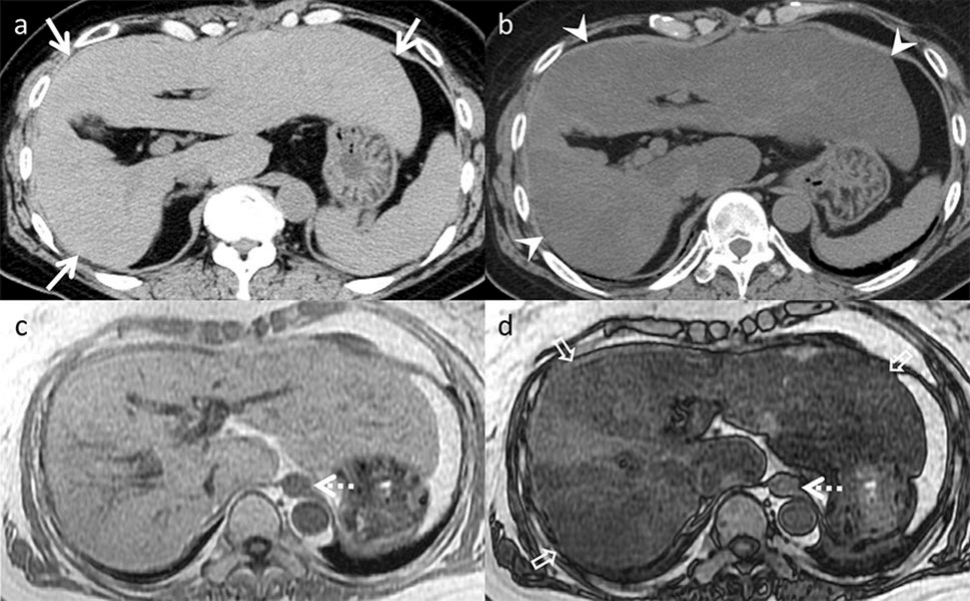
Chemotherapy-associated steatohepatitis in a 62-year-old woman with ascending colon cancer, who was undergoing chemotherapy (CTX), including irinotecan for left adrenal metastasis (dotted arrow). a Unenhanced computed tomography (CT) demonstrates normal liver attenuation (CT value, 55 HU) before CTX (arrows). b Decreased liver attenuation (21 HU) is observed on unenhanced CT performed seven months after CTX initiation (arrowheads). Additionally, compared to the c in-phase chemical shift imaging (CSI), a heterogeneous signal drop (open arrows) is seen on the d opposed-phase CSI. No abnormal liver function or hepatitis was indicated by hematological data or clinical symptoms. It is difficult to differentiate chemotherapy-associated steatohepatitis from simple liver steatosis on a single image alone
